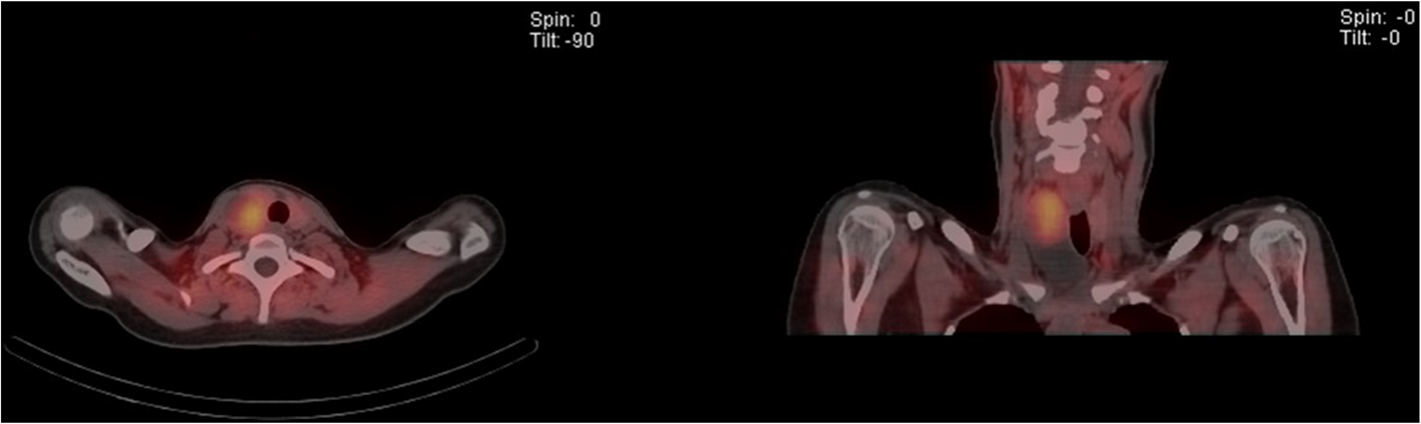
Single-photon emission computed tomography/computed tomography image at 2.5 hours post-radiotracer injection (Tc99m sestamibi) demonstrating a large lesion in the right lobe of the thyroid
